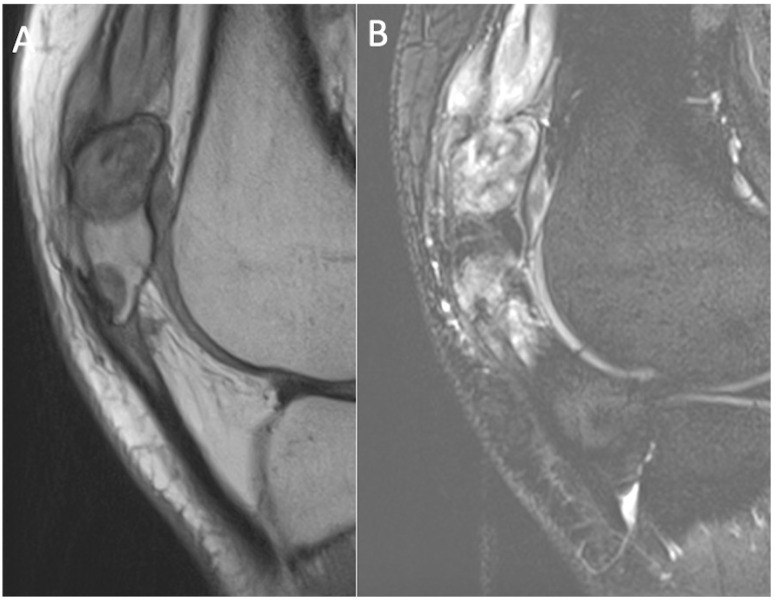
T1-weighted (A) and STIR (B) sagittal MRI demonstrating tophi at the distal quadriceps and proximal patellar tendons, with only mild oedema in the adjacent bone.
